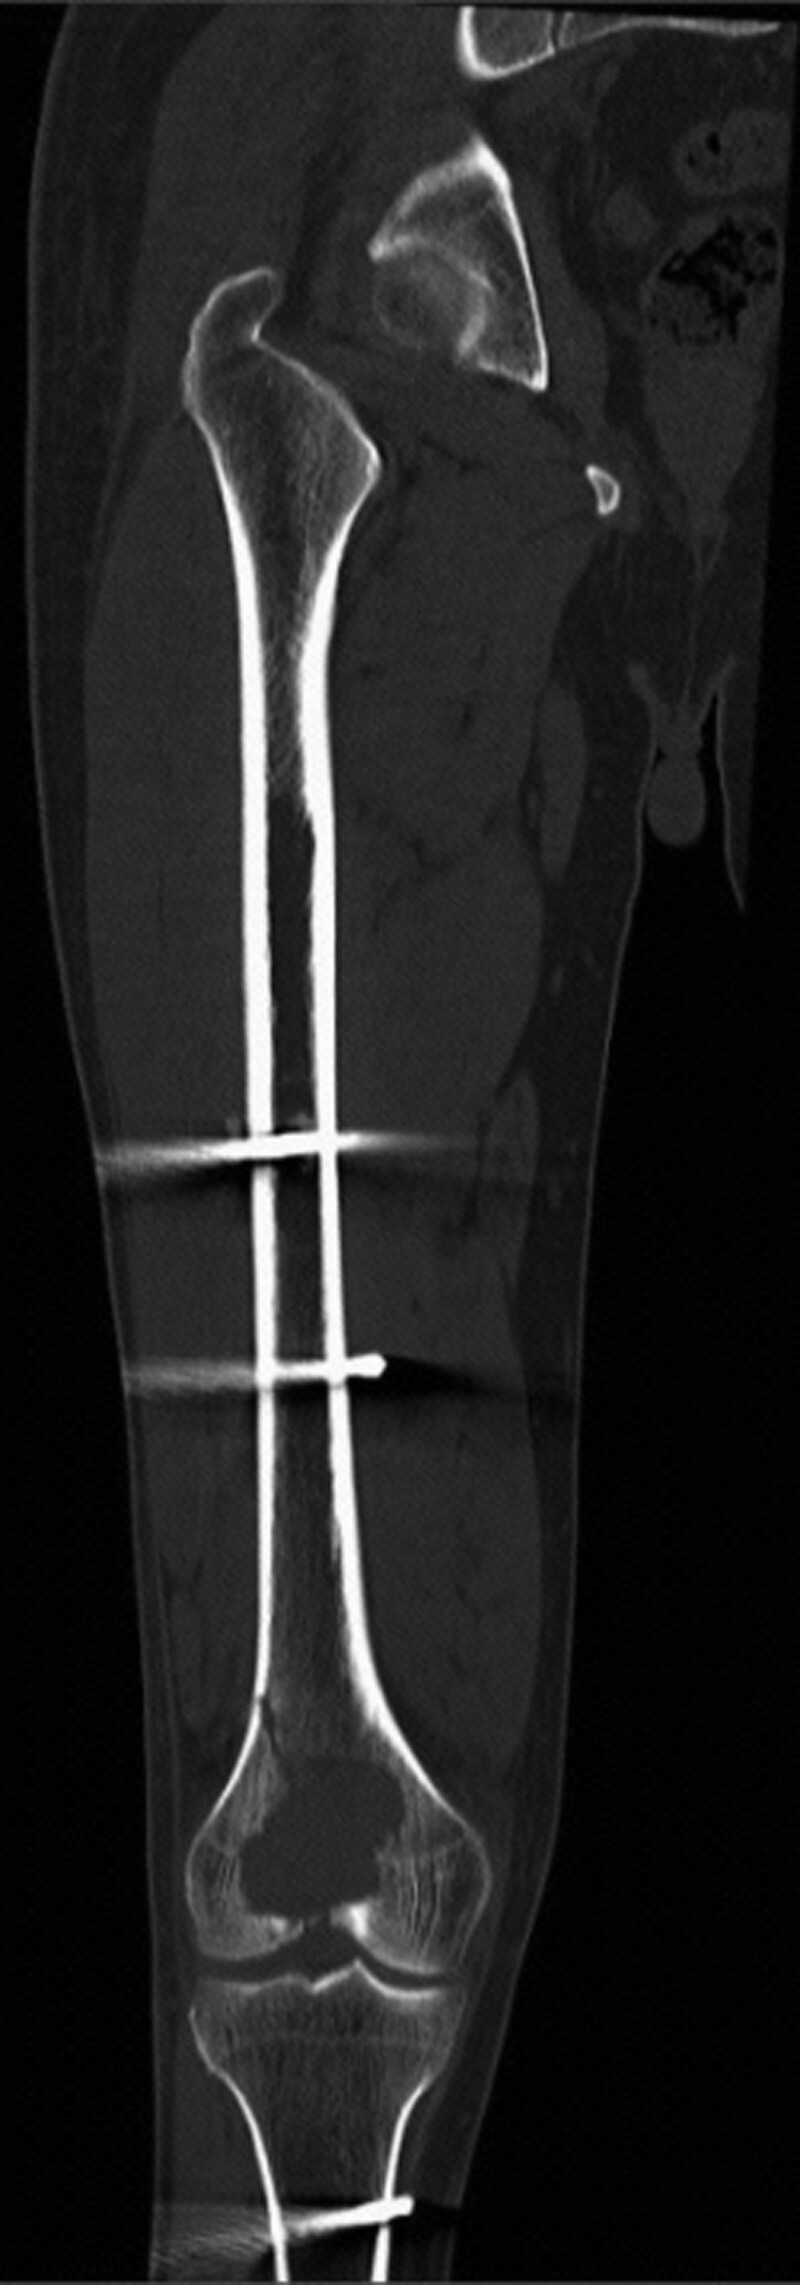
Plain scan CT showed an expansile and well-defined bone destruction in the right distal femur, without calcification, ossification shadow or sclerotic border. Multiple fracture lines were seen around.
